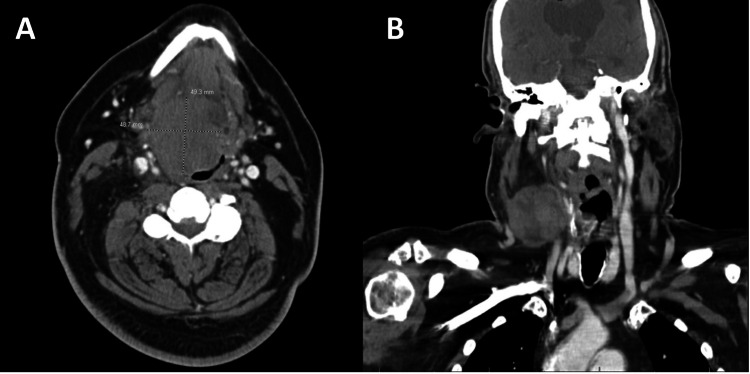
Contrast-enhanced computed tomography (CT) of the neck (A) Large right tongue base mass largely obliterating the oropharyngeal airway. (B) Prominent necrotic lymph node noted in the right level II/III of the neck.
